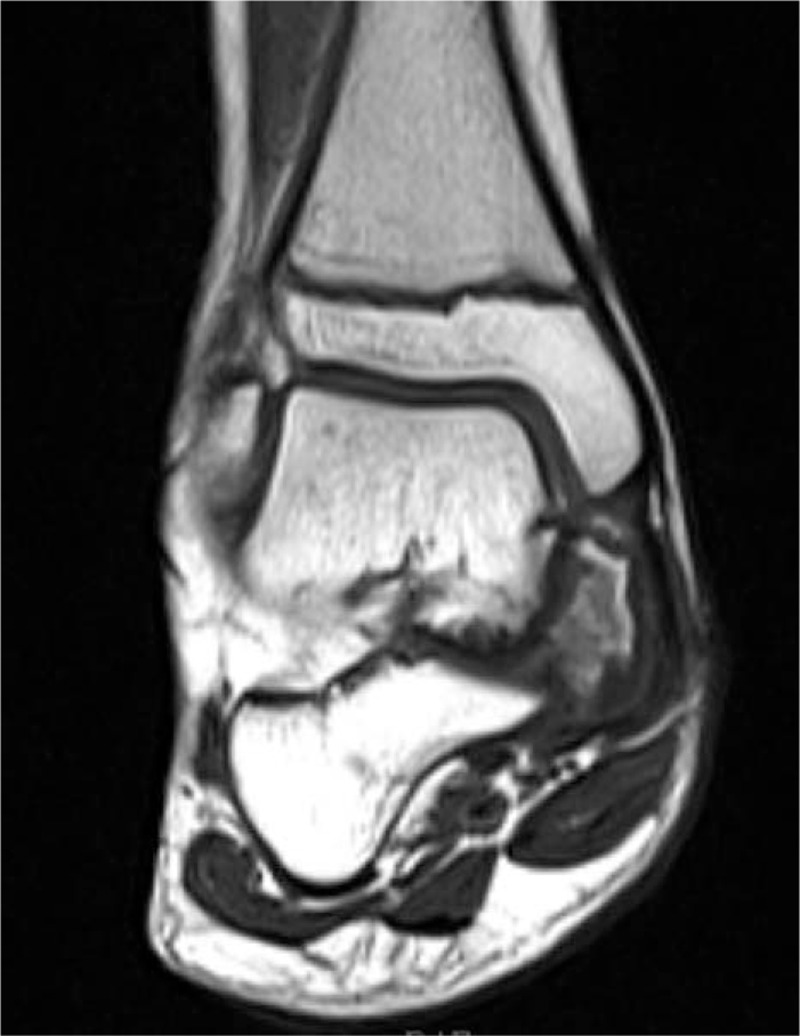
MRI showed a free mixed signal lesion and obvious sclerosis in the talus process and medial talus of the right foot root.
